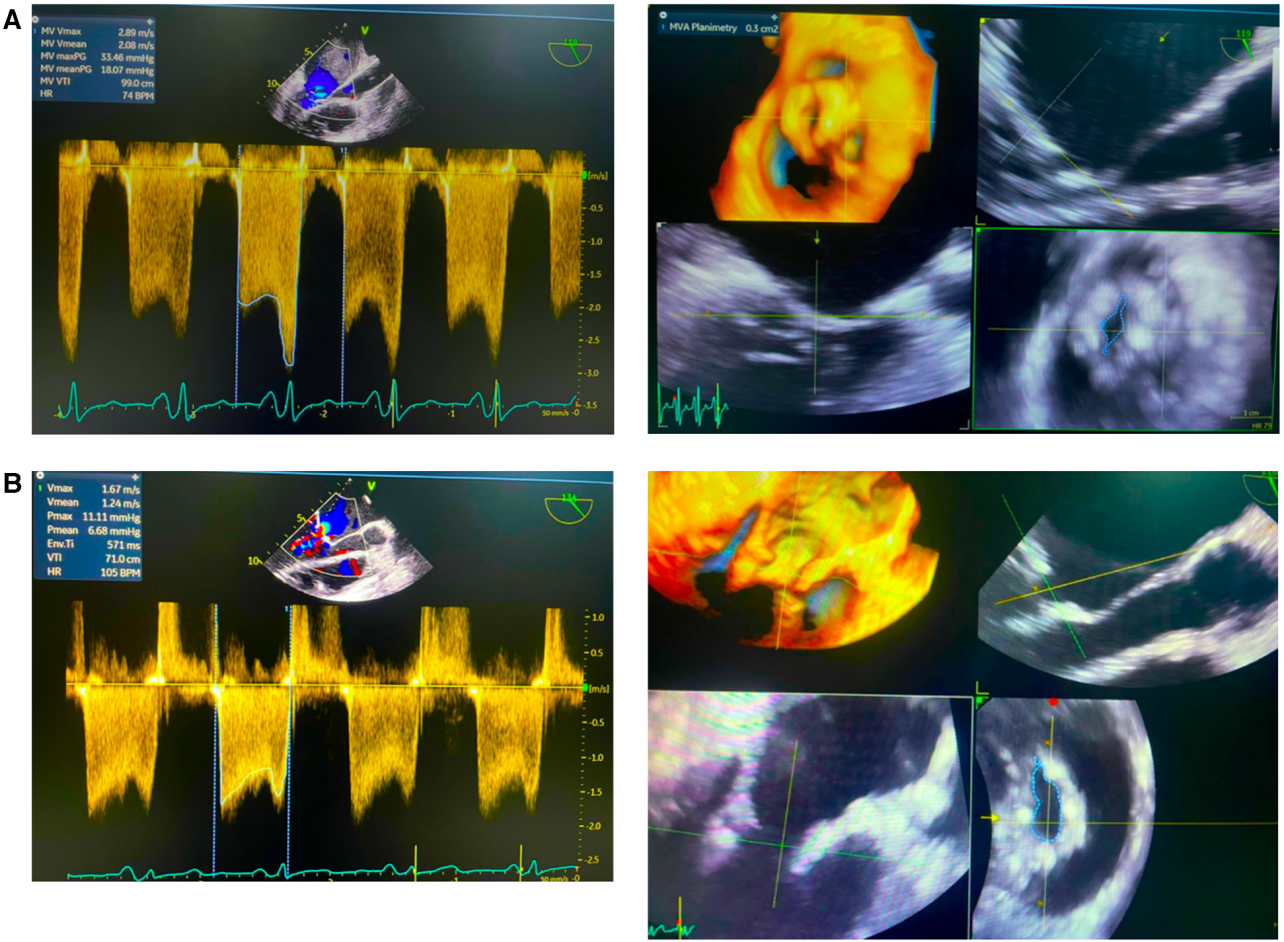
Echocardiography pre- and post- percutaneous balloon mitral valvotomy (PBMV). (A) Initial data showed mean valve area (MVA) plannimetry was 0.3 cm and mean valve gradient (MVG) 18.7 mmHg. (B) Post PBMV showed mean valve area (MVA) plannimetry was 1.0 cm and mean valve gradient (MVG) 6.68 mmHg.
